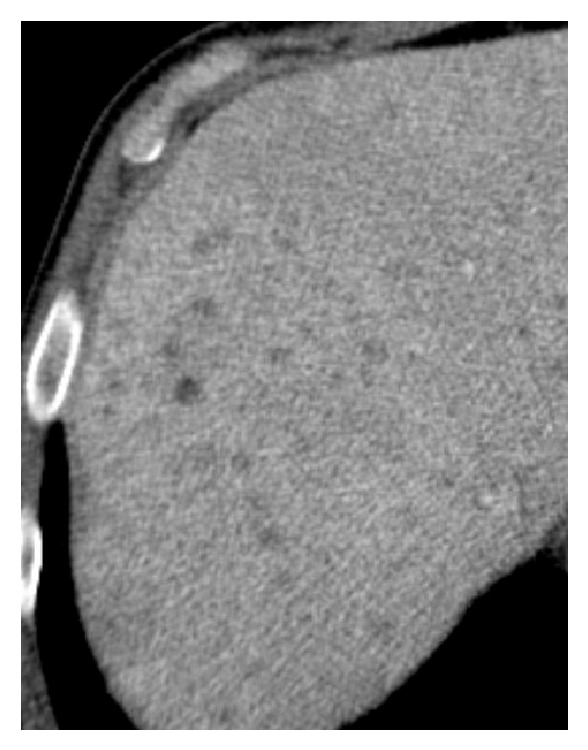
Magnification of axial contrast-enhanced abdominal CT: liver nodules can be best appreciated, some confluent. Note the hypodense area (fat attenuation values) of one of the nodules, indicating xanthomatous type lesion.
